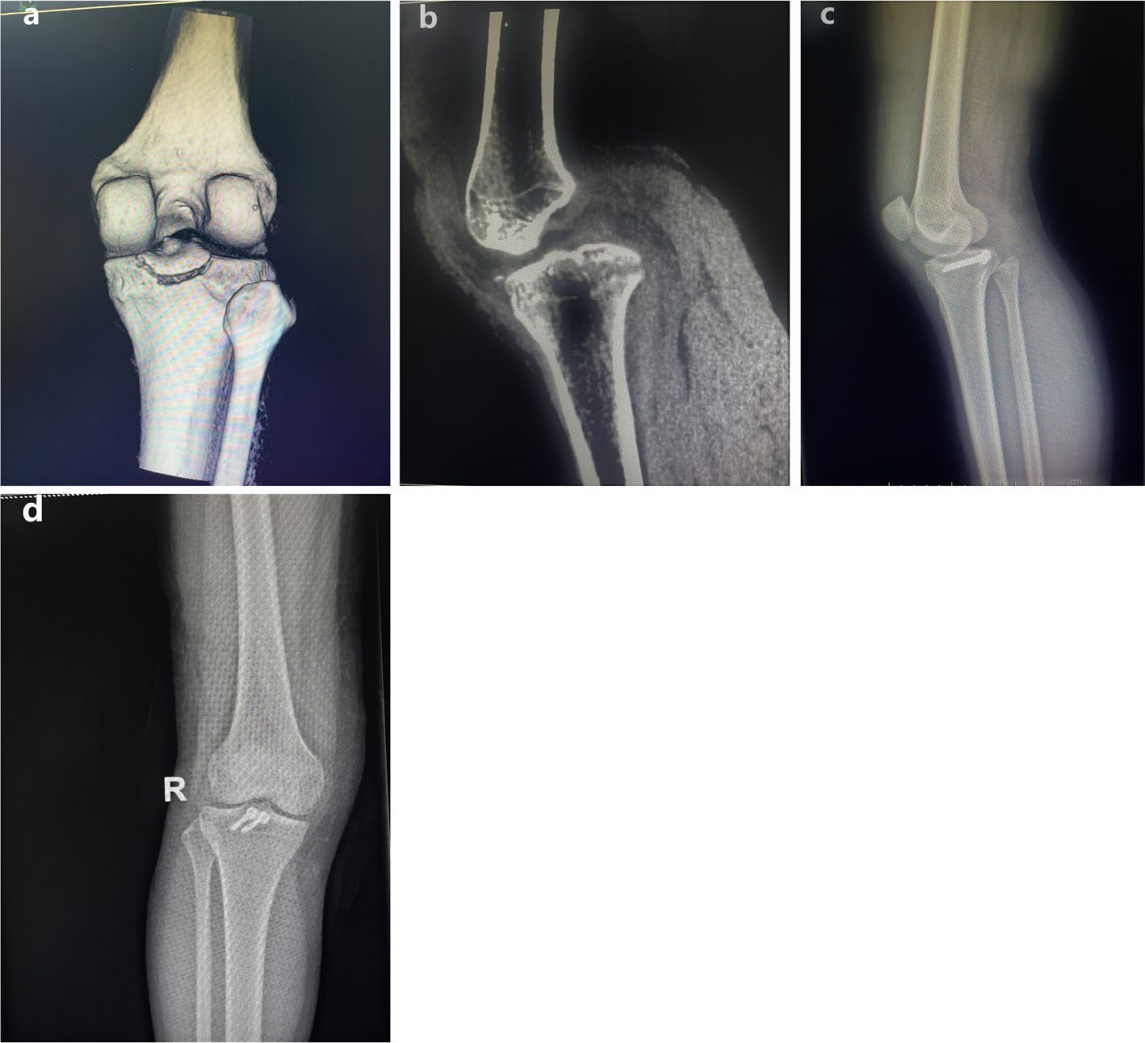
a,b Preoperative CT showed an avulsion fracture of the posterior cruciate ligament. c,d A hollow lag screen combined with a gasket operation. X-ray films of the patient’s knee joint on the 2nd day after the operation
